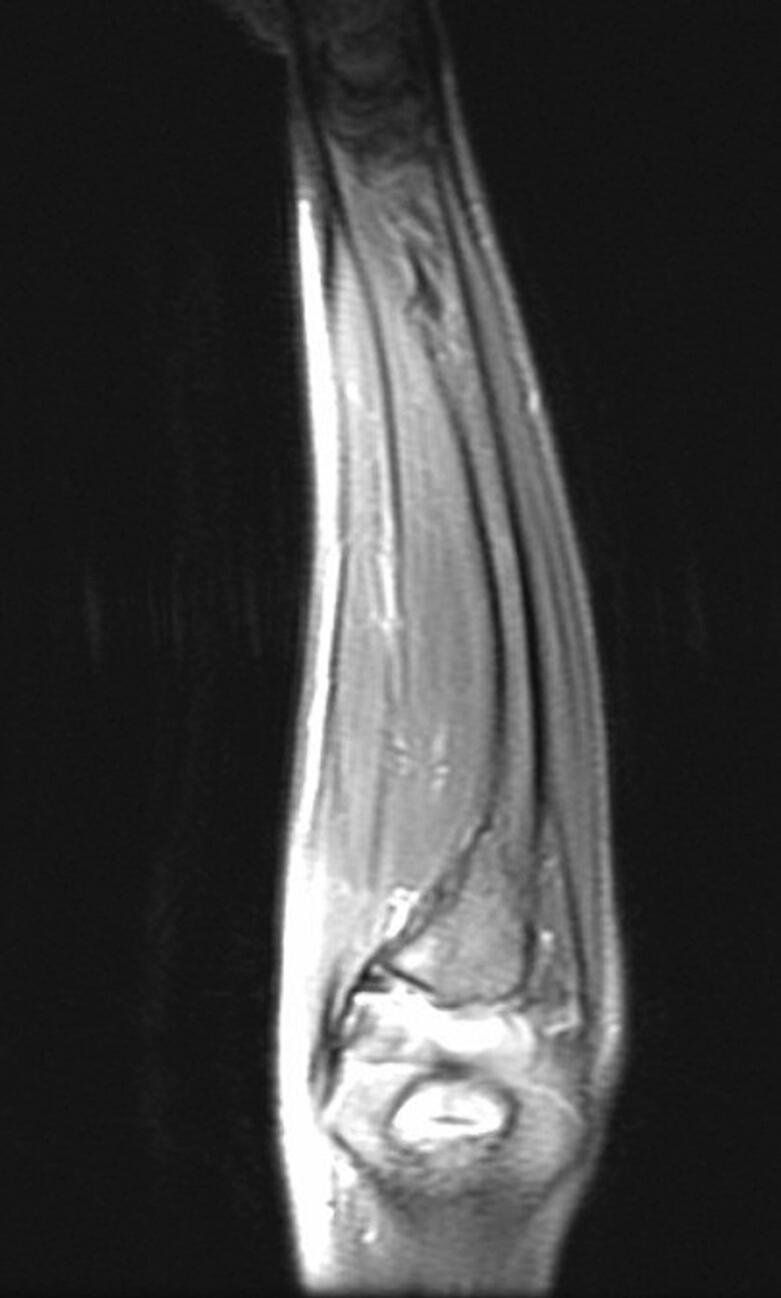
Preoperative MRI indicates the FCD at the distal ulna (sagittal view).
